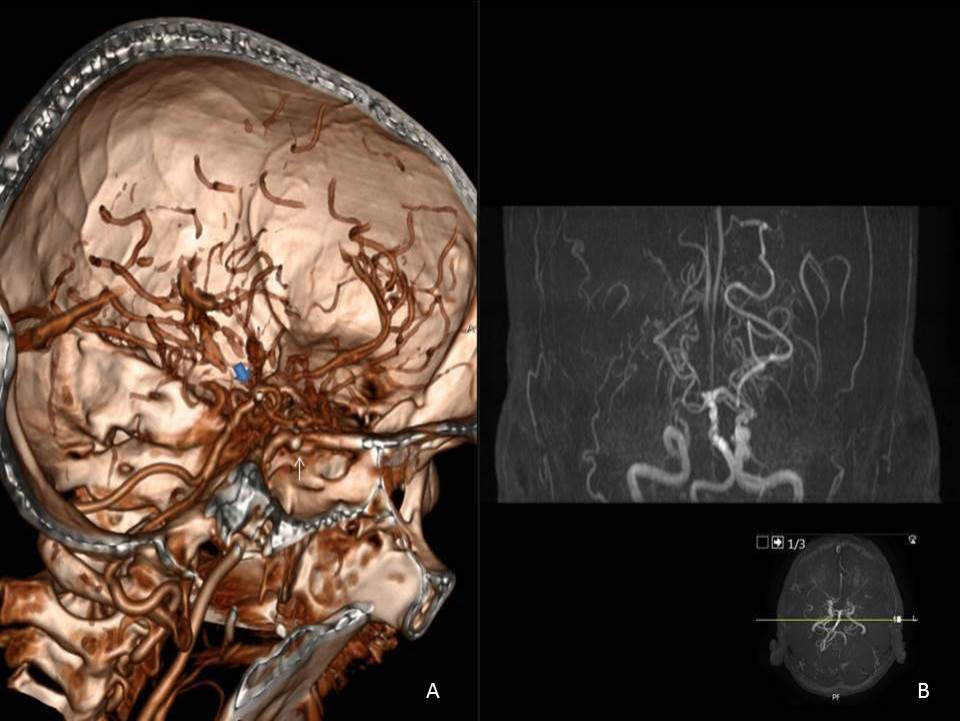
(A) Contrasted computed tomography scans of cranium revealing internal carotid artery occlusion at the level of supraclinoid segments (white arrow) and opacifications of distal segments of bilateral anterior cerebellar and middle cerebellar arteries (blue arrow); (B). Abnormal coronary collaterals (puff of smoke sign).
